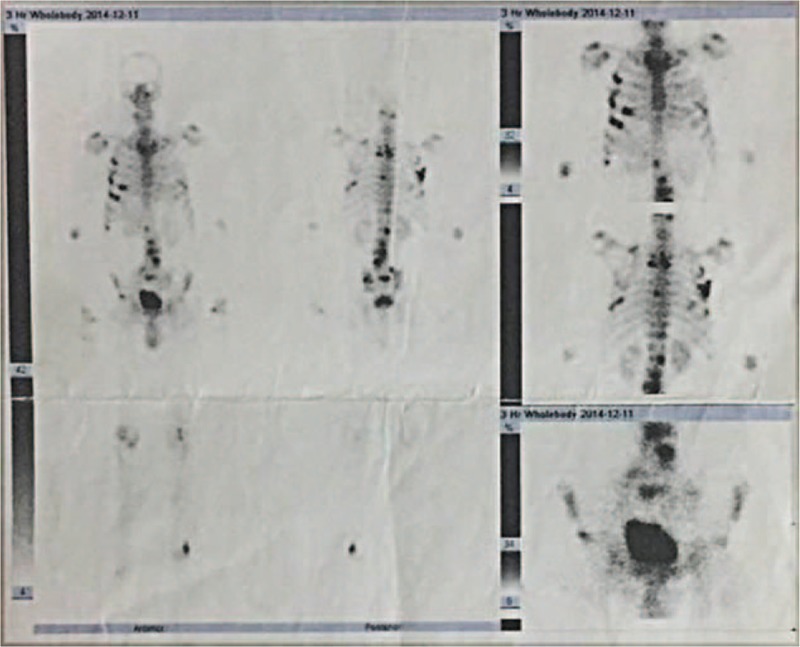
SPECT/CT scans show multiple bone metastases.
